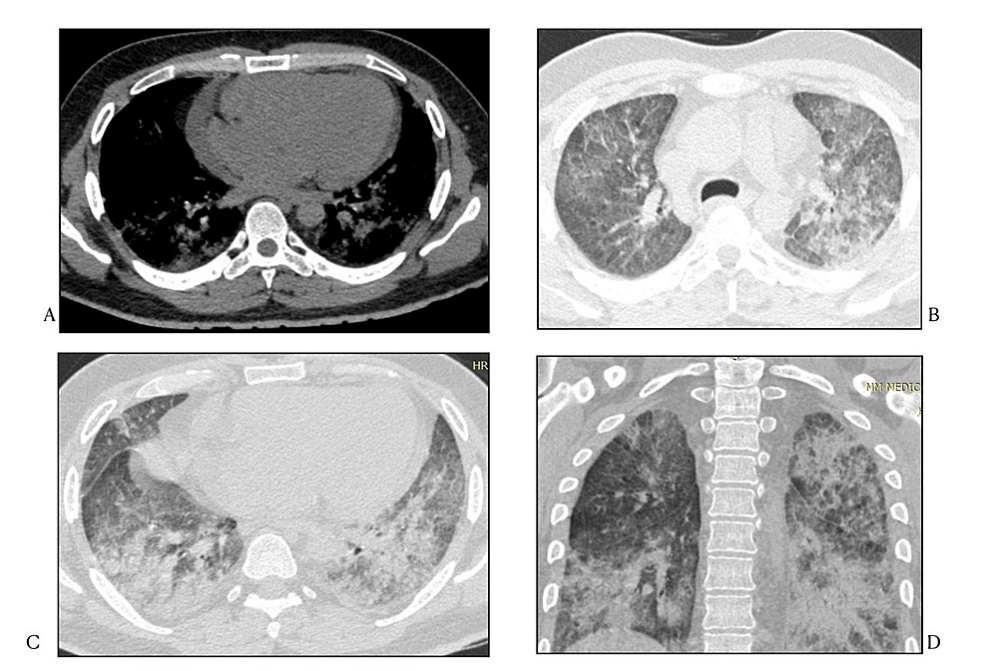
Patient with CT severity score of 21/25 HRCT CHEST (mediastinal and lung windows) axial and coronal reformatted images show diffuse changes of acute respiratory distress syndrome with cardiomegaly and pericardial effusion
